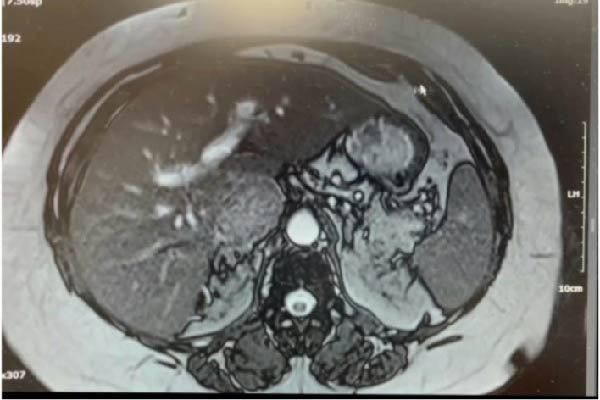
Cross‐section MRI showed lesion in IVC.
